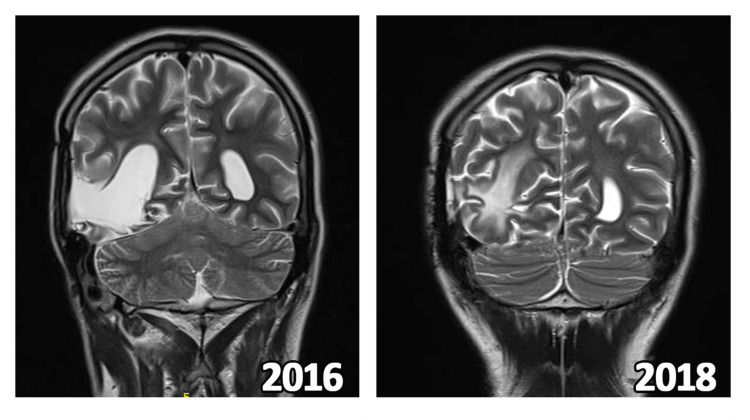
MRI of Patient 2 during various phases of follow up in 2016 and 2018
